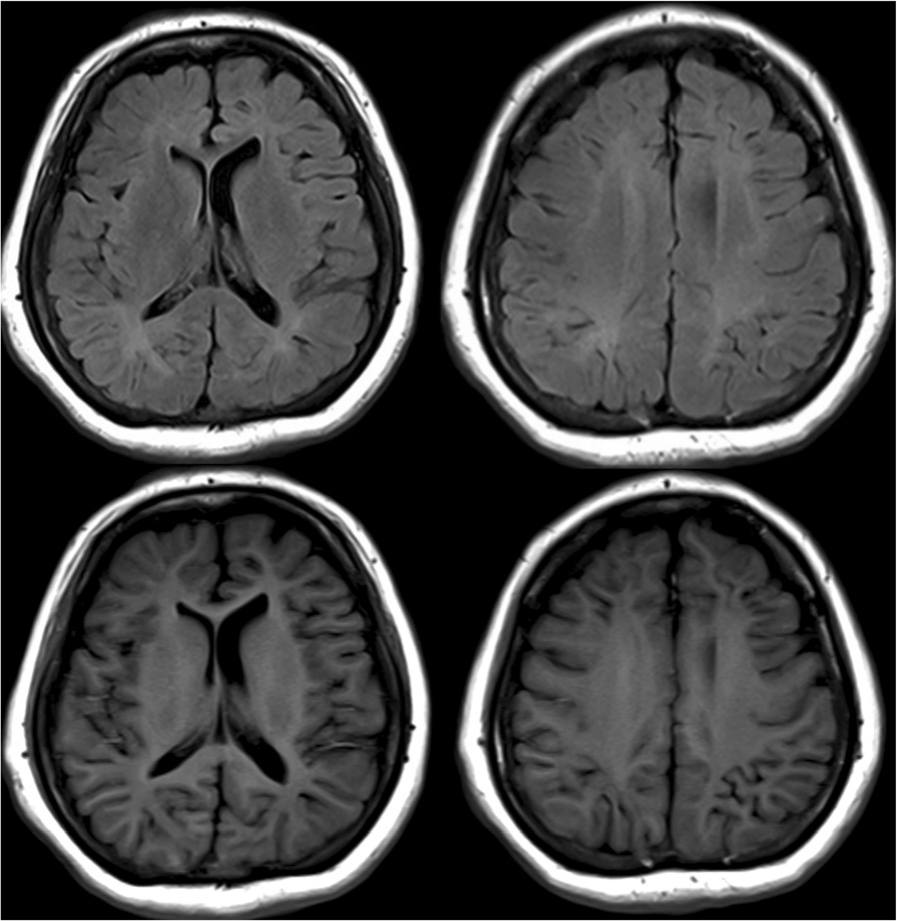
Brain magnetic resonance imaging. Axial T2-weighted fluid-attenuated inversion recovery image (above) and T1-weighted image (below) at different level showed prominent smaller right lateral ventricles. Diffuse periventricular and subcortical white matter hyperintensities are also noted.
